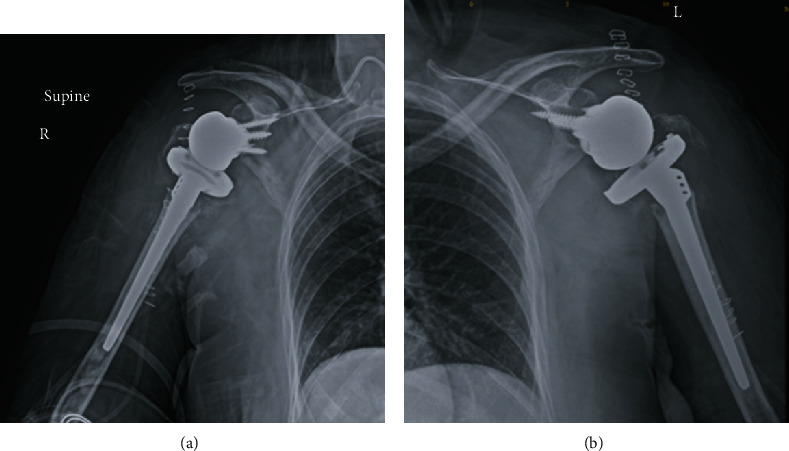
(a) Postoperative X-ray radiograph of the right shoulder showing reverse shoulder arthroplasty. (b) Postoperative X-ray radiograph of the left shoulder showing reverse shoulder arthroplasty.
